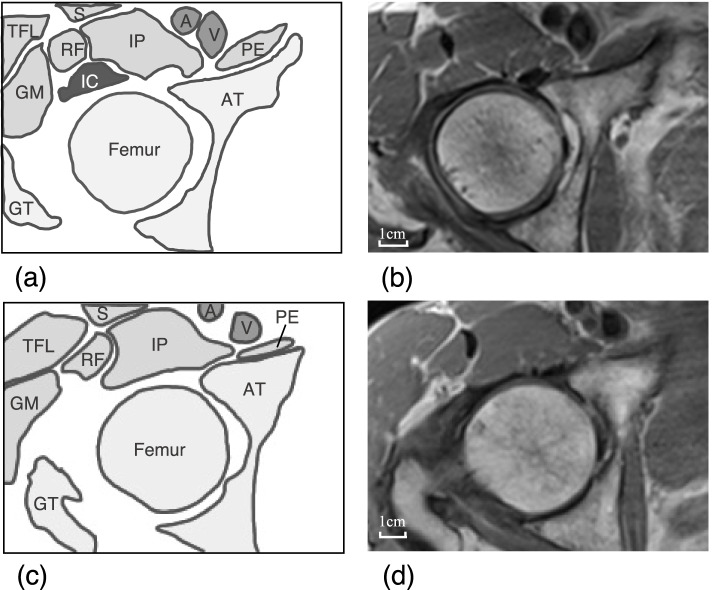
Examples of MRI images from two representable individuals. a and b were imaged from an individual who can be observed IC. c and d were imaged from another individual who cannot be observed IC. This figure was developed by referring to a previous study [6]. GM; Gluteus minimus, IC; Iliocapsularis, IP; Iliopsoas, PE; Pectineus, RF; Rectus femoris, S; Sartorius, TFL; tensor fasciae latae, Femur; femoral head, GT; greater trochanter, AT; acetabular, A; femoral artery, V; femoral vein
